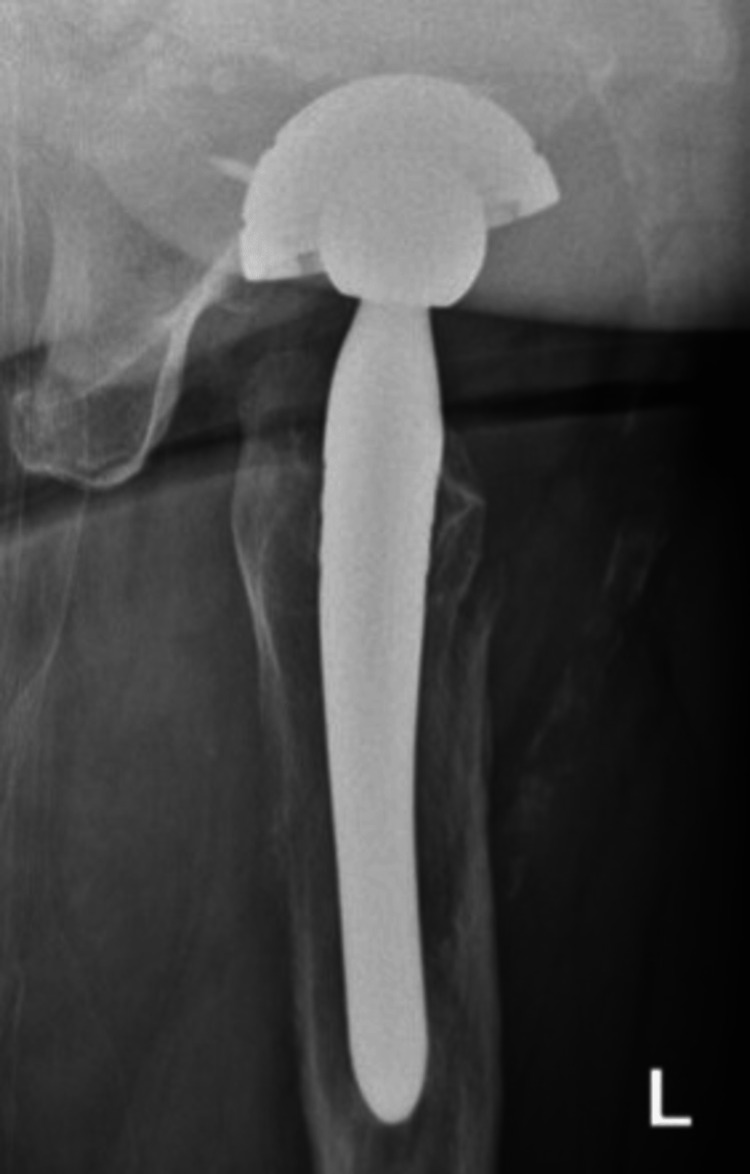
Case C, left X-hip-axial pre-OR X-ray showing a periprosthetic femoral fracture of the left femur, Vancouver B2 type of fracture
